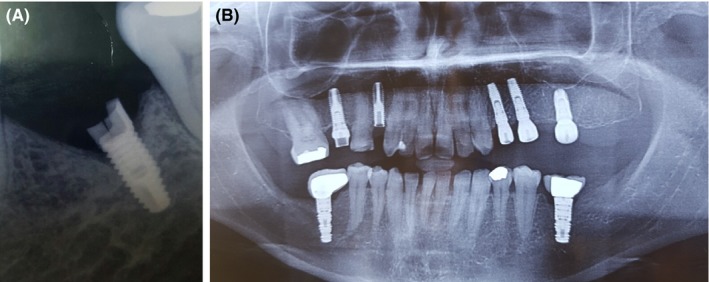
A, Periapical radiograph showing fractured piece stuck in fixture. B, Orthopantomograph showing fractured screw fixture
